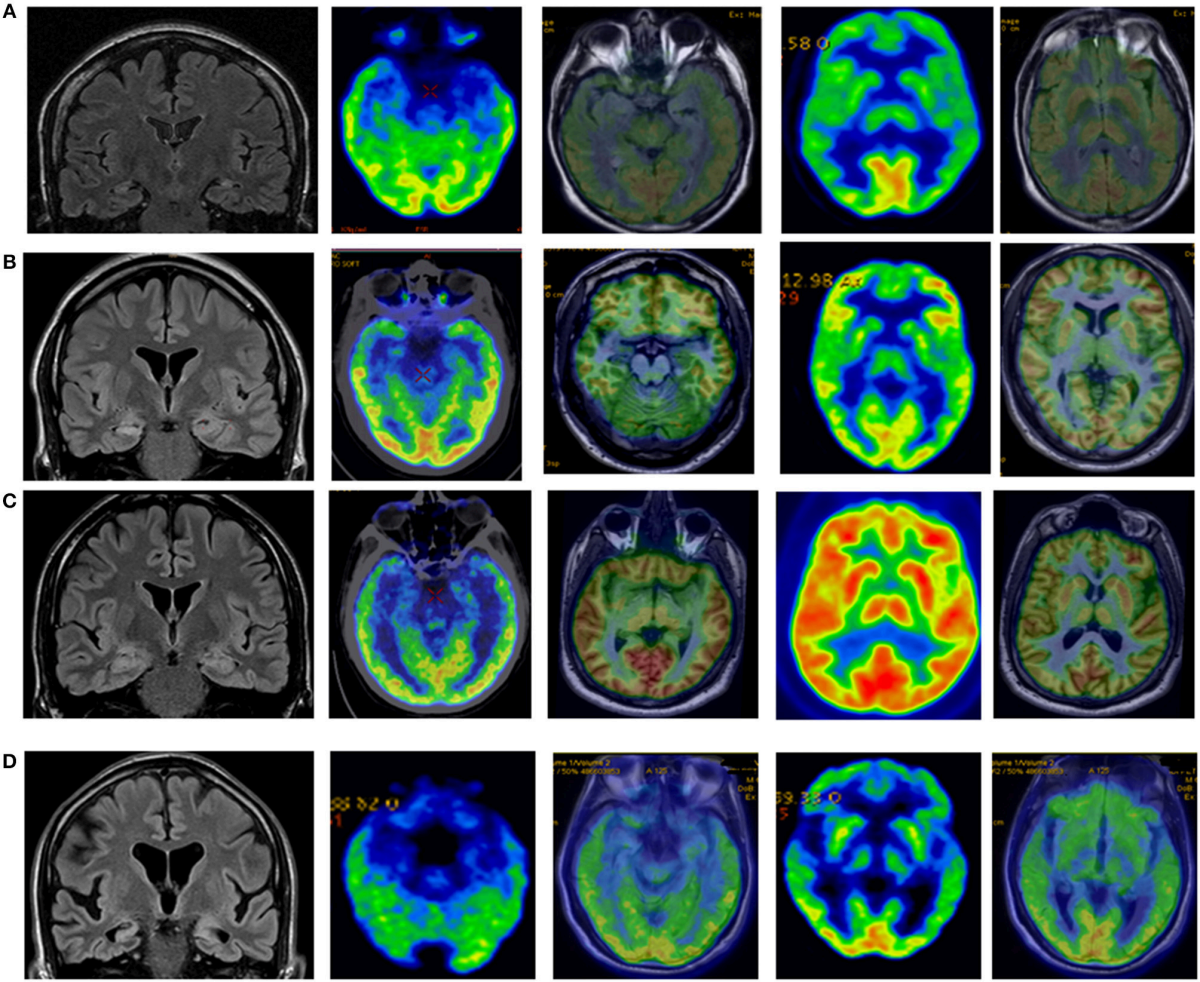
(A) Control patient who suffered measles encephalitis at 2. Epilepsy started at 8. Currently (more than 20 years later) suffers weekly seizures and severe memory deficits. MRI shows bilateral hippocampal atrophy and FDG PET bilateral medial temporal lobe hypometabolism with normal insular metabolism. (B) Control patient who suffered febrile seizures. Epilepsy started at 9. He suffered weekly FIAS preceded by non-specific sensation. MRI right hippocampal hyperintensity and FDG-PET right mesial hypometabolism with normal insular metabolism. (C) Patient with T1DM from age 29 and temporal lobe epilepsy from age 30. MRI remains normal after 5 years but FDG-PET shows bilateral medial temporal lobe hypometabolism and left insula hypometabolism. (D) Patient with epilepsy from age 56. Seizure-free but progressive and severe memory decline. MRI shows bilateral hippocampal sclerosis and insular atrophy, and FDG-PET shows bitemporal medial hypometabolism and bilateral insular hypometabolism.
